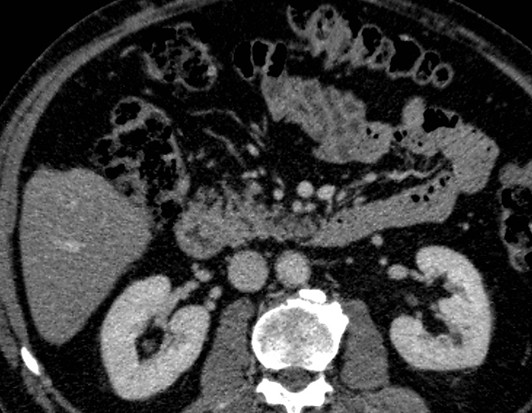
CT follow up examination. It shows the patient free of the disease.
